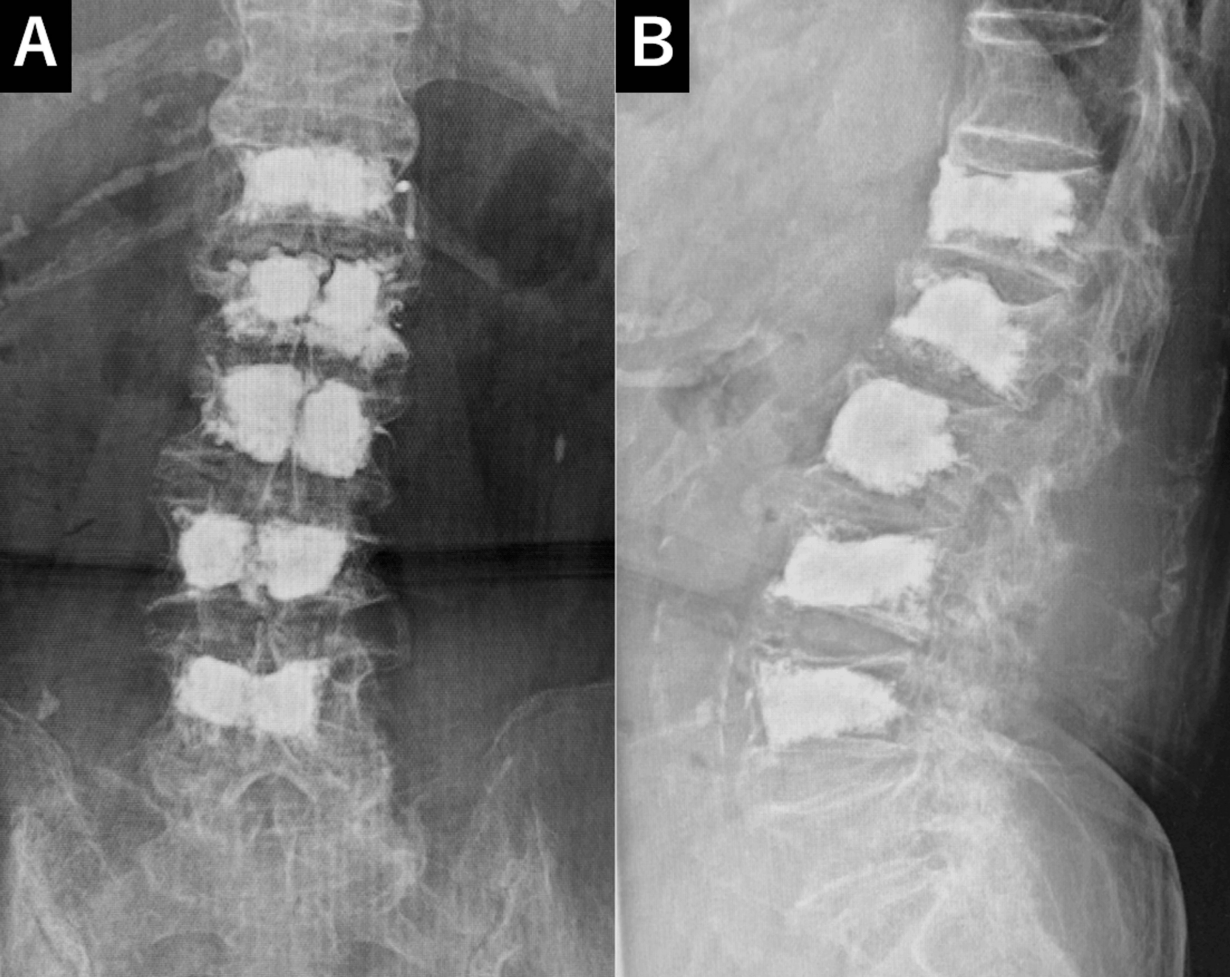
X-ray one year after BKP No new vertebral compression fractures are observed, and spinal alignment is maintained. BKP: Balloon kyphoplasty
